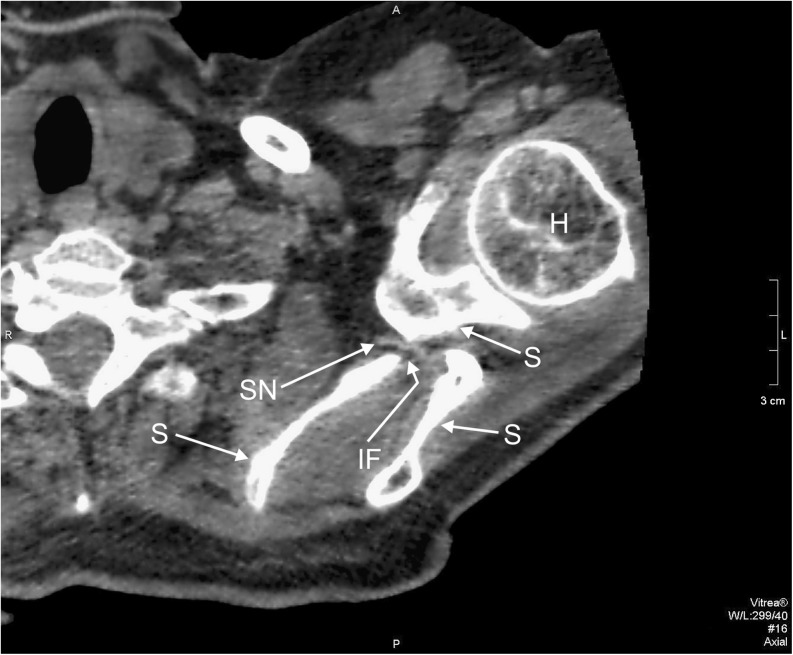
Dual-phase helical CT, transverse scan on the level of the inferior suprascapular foramen: *H* humerus, *IF* inferior suprascapular foramen, *SN* suprascapular nerve, *S* scapula
